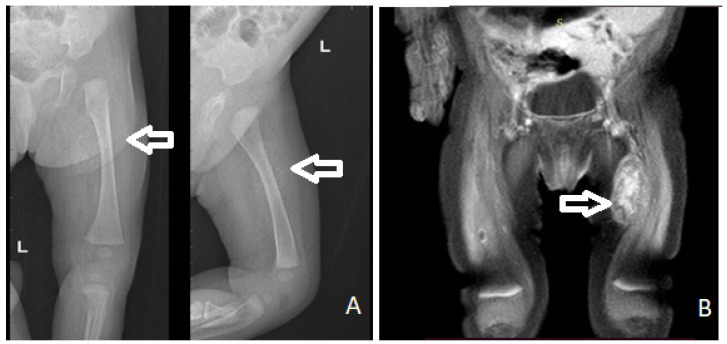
X-ray (A) and MRI (B) showing a mass on the antero-intern part of the left thigh (arrow).
